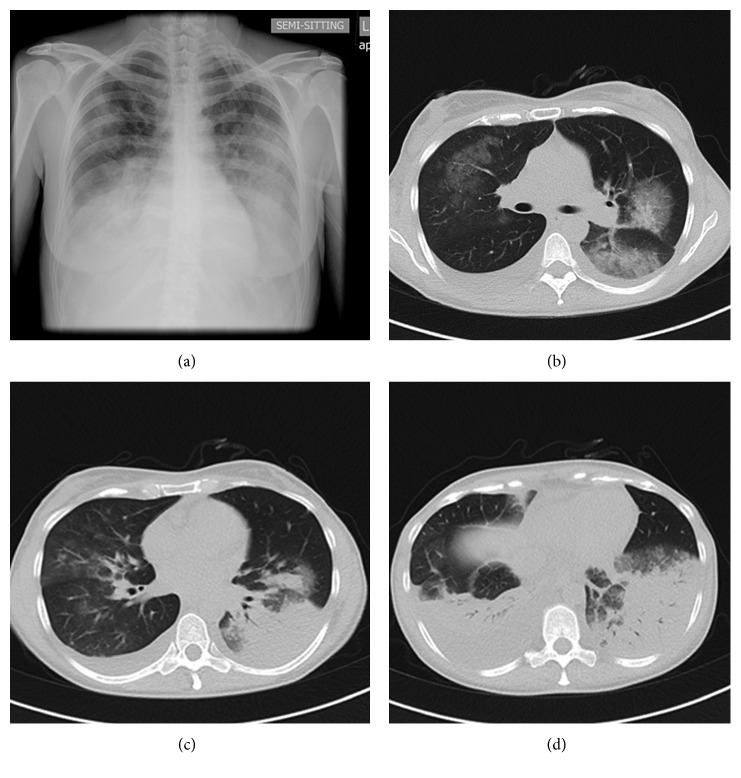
Chest CT scan and chest X-ray (semisitting position, posterior-anterior view) of the patient after clinical deterioration depicting diffuse bilateral opacities.
